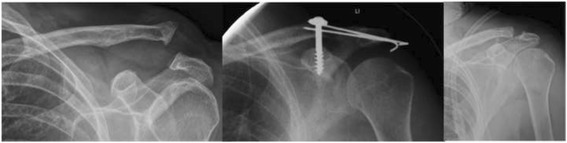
Shows an exemplary case of a Rockwood IV AC dislocation; initial injury X-ray; X-ray after treatment with Bosworth screw and K-wiring; and final result after removing the implants
